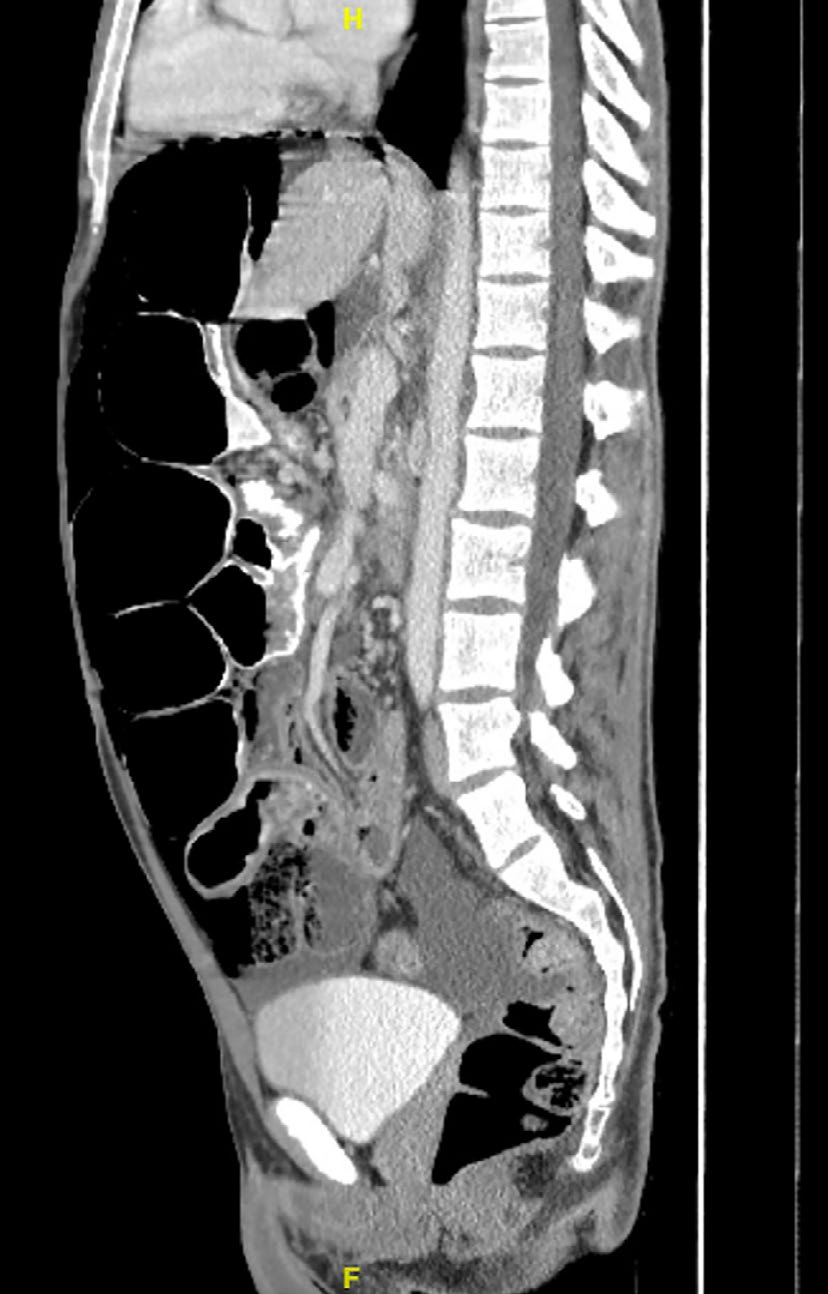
Sagittal cross‐section of CT abdomen and pelvis revealing distended loops of bowel. H: toward patient head and F: toward patient feet.
